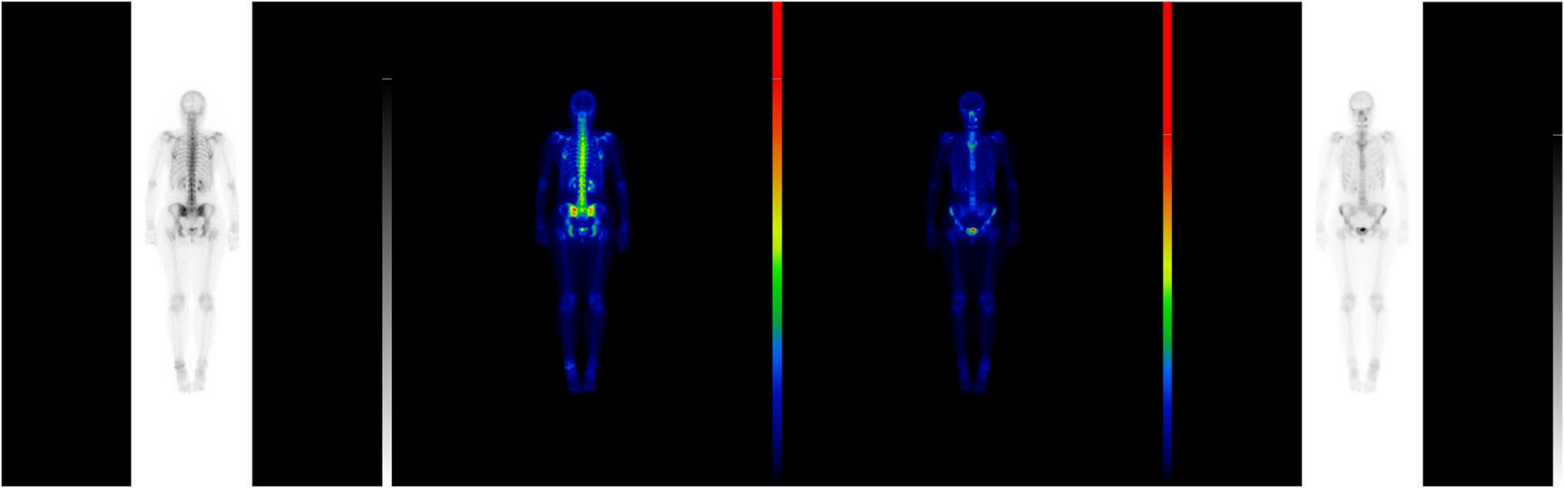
Bone scintigraphy (Tc99m MDP): increased tracer accumulation in the posterior part of the right rib 11
